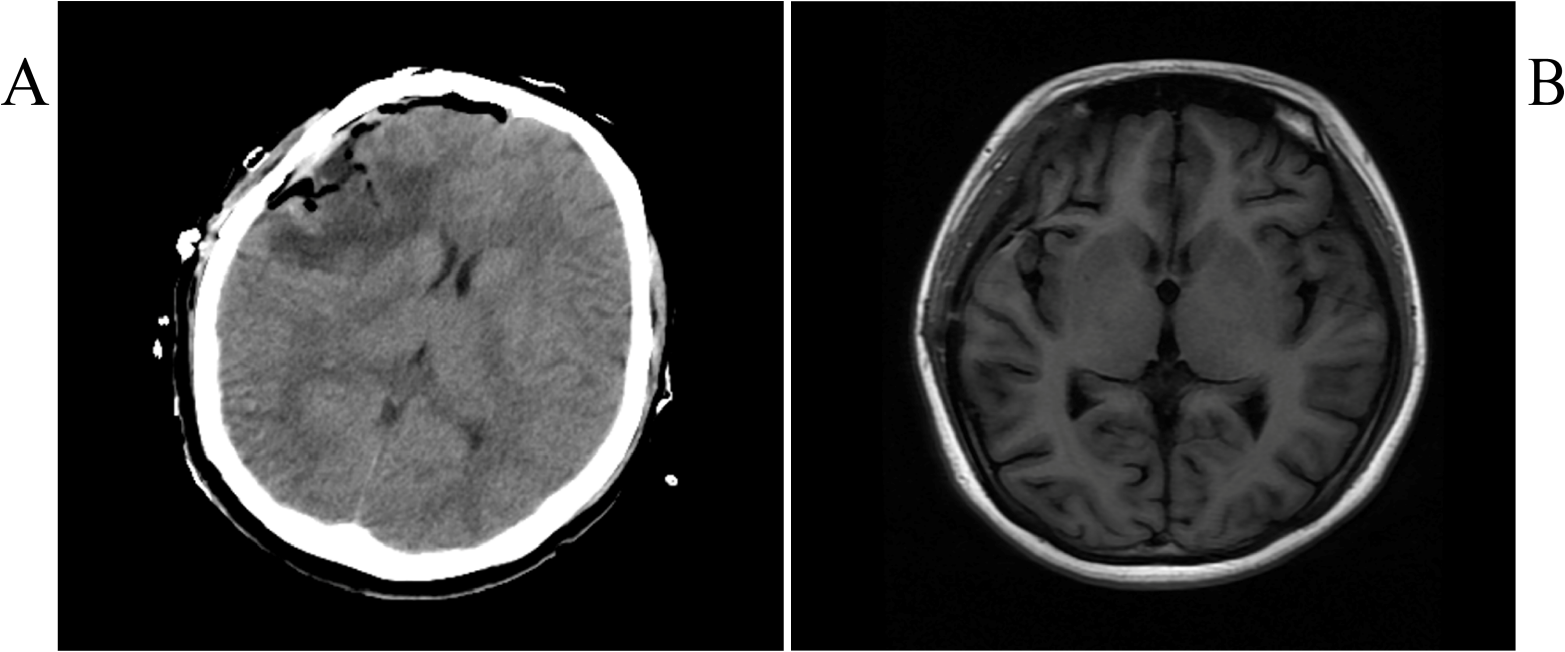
Postoperative CT (A) and MRI (B).
